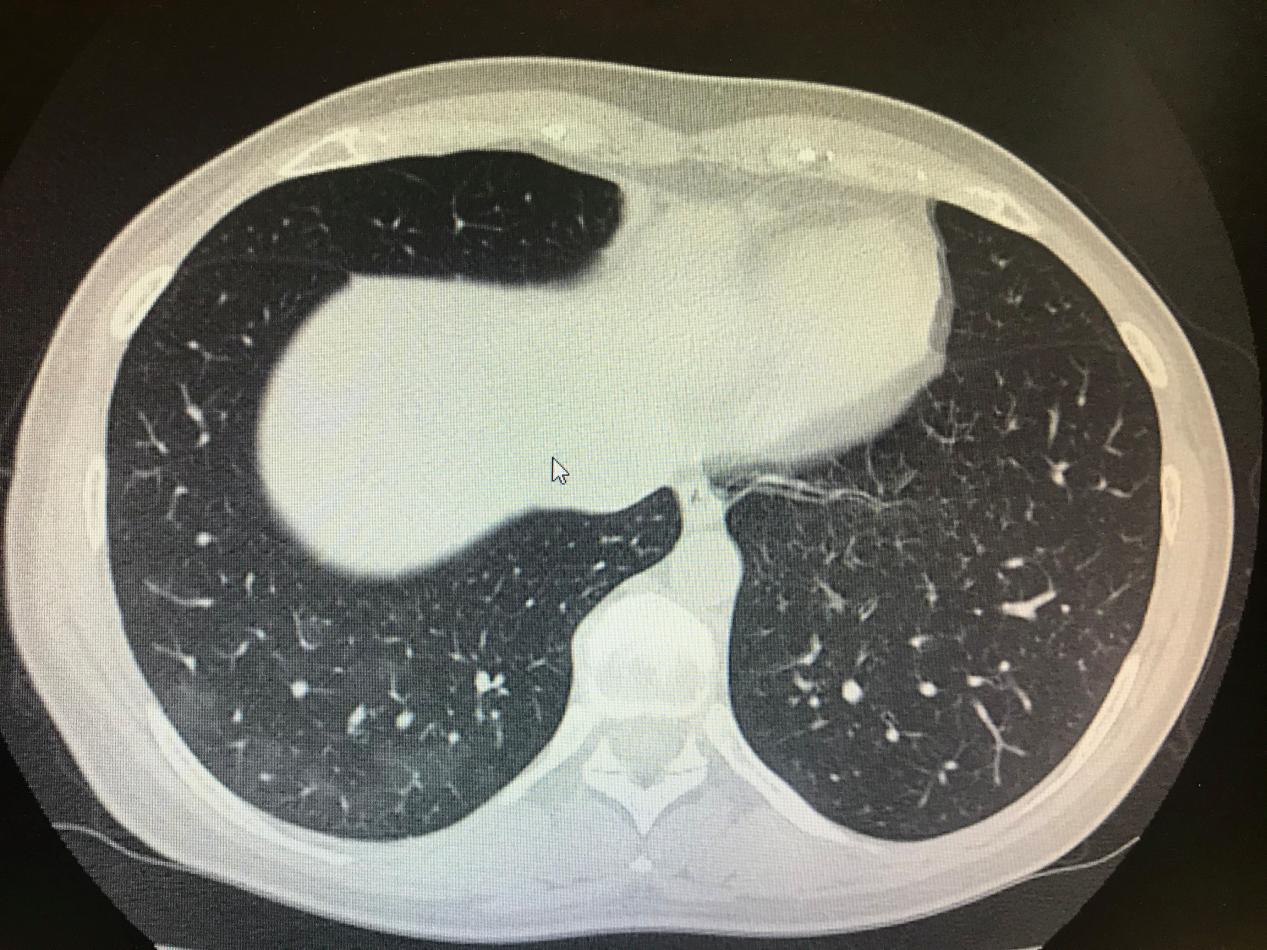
Lung CT scan after treatment with pneumonia resolution.
